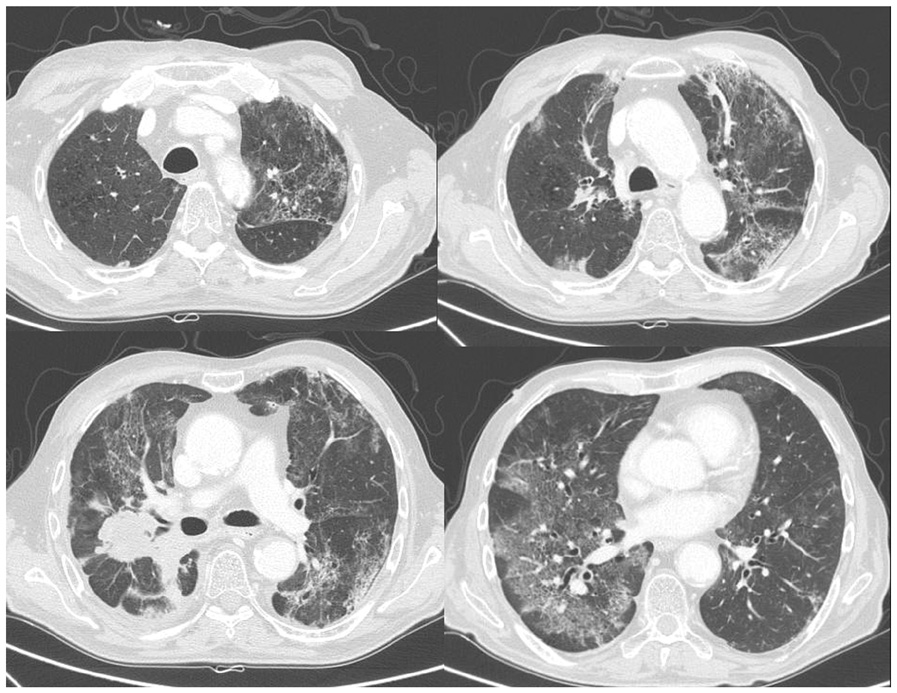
CT scan images observed 4 months after the beginning of radiotherapy, in one of the three patients with a strong and sustained increase in FeNO measured during radiotherapy.
